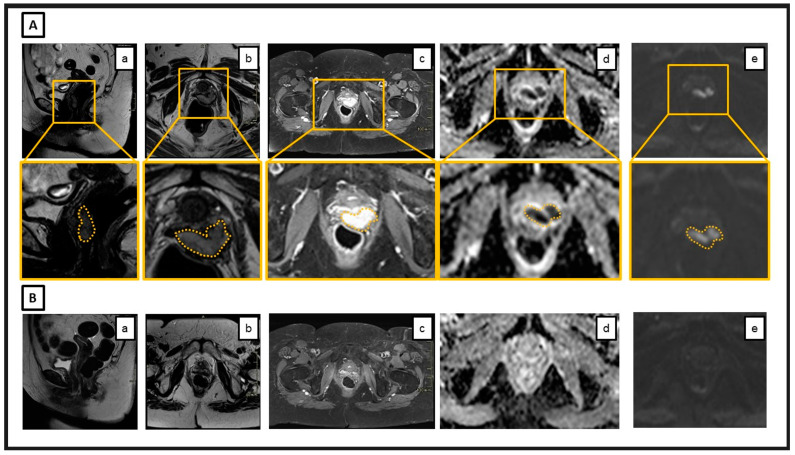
The panel (A) shows baseline magnetic resonance images (MRI): a lesion originating from the left anterior vaginal wall can be observed, involving the middle–lower third of the vaginal canal and extending beyond the paracolpium in the left anterolateral area. This lesion shows an intermediate signal on T2 (a,b), with homogeneous and regular contrast enhancement (c), and marked signal restriction on diffusion sequences (d,e). For each sequence, an enlargement of the disease (outlined in ochre) is provided. In panel (B), the MRI images correspond to the post-CIRT follow-up and document a complete response to the treatment. Specifically, the previously described tissue in the vagina is no longer recognizable (a,b). There are no areas of pathological contrast enhancement (c) nor signal restriction on the diffusion sequences (d,e).
